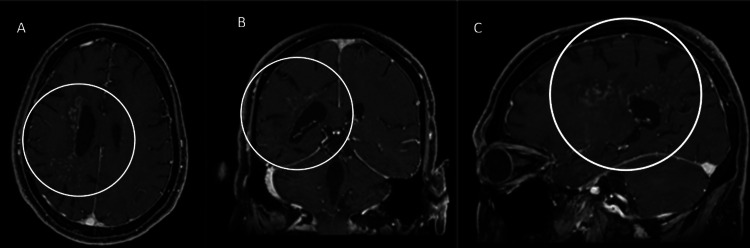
Five-month follow-up enhanced MRI At five months post-treatment, the patient's enhancement patterns show curvilinear and nodular enhancement speckled throughout the white matter. It predominates in a perivascular and periventricular distribution. The axial (A), coronal (B), and sagittal (C) planes are depicted with the abnormal enhancement marked by the unfilled circles.
